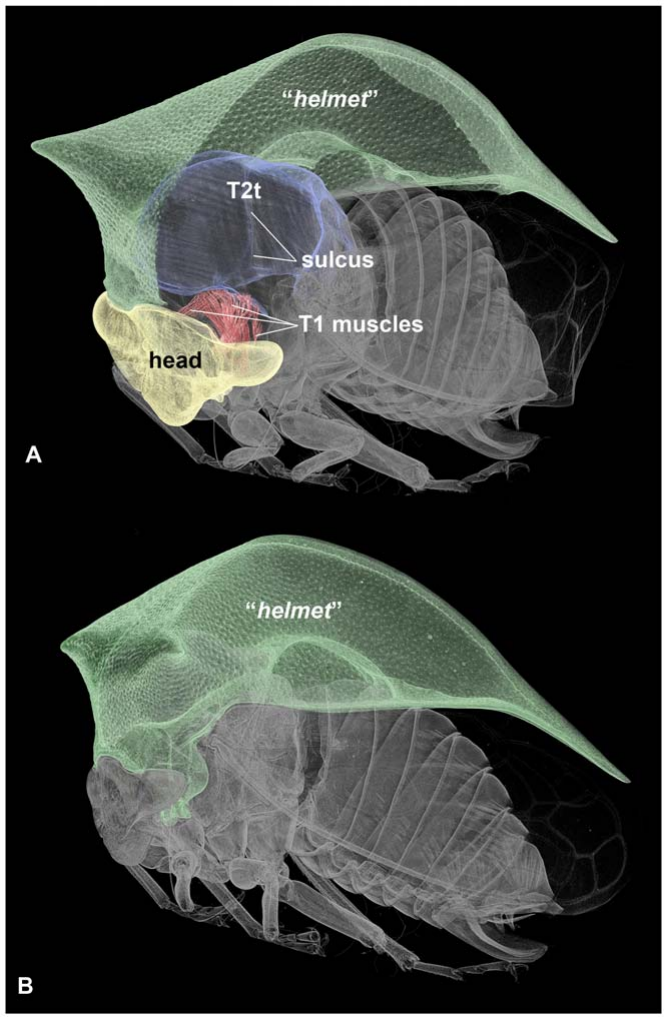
Micro-computed tomography of *Stictocephala bisonia* (Membracidae), showing the relationship between the “*helmet*” and other anatomical structures (volume renderings of μ-CT data). A: Habitus, anterolateral view, left half of “*helmet*” removed. Head, T1 muscles, T2 tergum and “*helmet*” are annotated by overlays. B: Habitus, lateral view, “*helmet*” is annotated by overlay. Abbreviation: T2t = T2 tergum.
